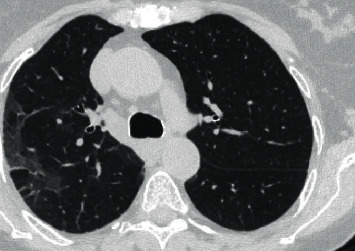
Repeat chest CT at three months showing interval improvement in right lobe infiltrate.
